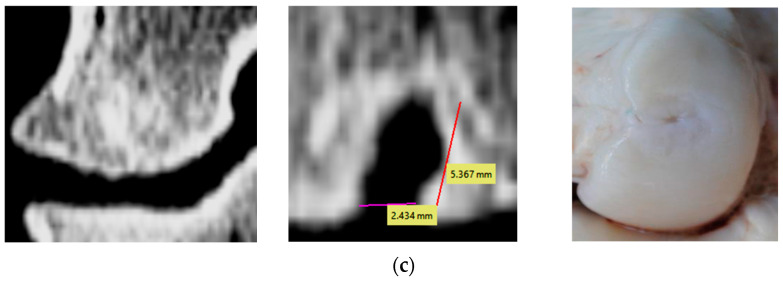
Comparative post-operative CT images of surgical treated areas (coronal and sagittal views): (a) Case 1 (just porous collagen); (b) Case 2 (Collagen + BMC); (c) Case 3 (Collagen + ASC).
